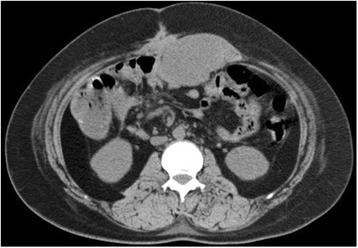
Computed tomography image of the abdominal wall mass
